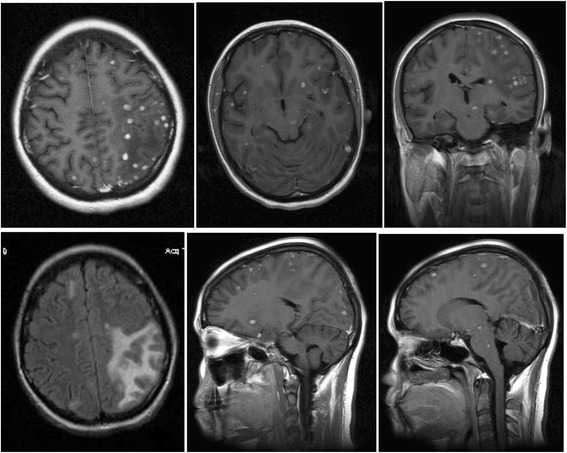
First magnetic resonance imaging brain images showing multiple intracerebral tuberculomas
